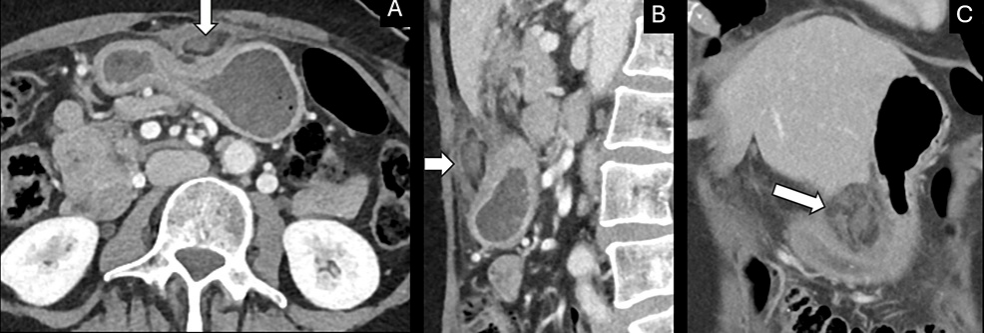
Lesser omental infarction. Axial (A), coronal (B) and sagittal (C) post-contrast CT images demonstrate a focal region of inflammation centered in the fat of the lesser sac/omentum.
